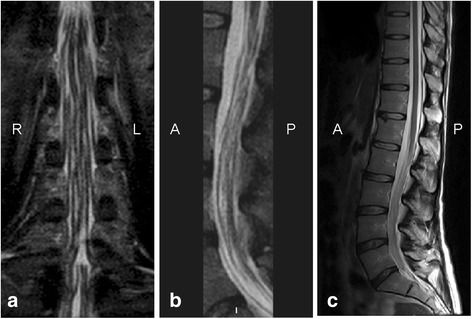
Spinal MRI study. MR coronal STIR (a), sagittal STIR (b) and T2-weighted sagittal (c) images of the cauda equina showing marked thickening of the nerve roots together with a tube-shaped enlargement. (R = right; L = left; A = anterior; P = posterior)
